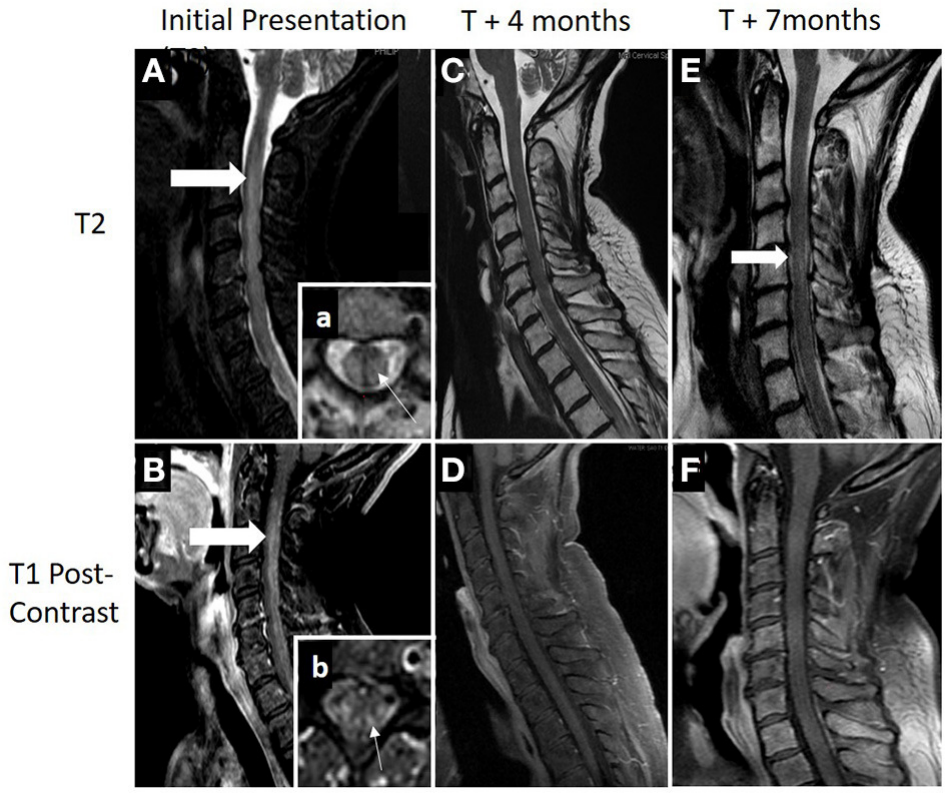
(A,B) MRI demonstrating an longitudinally extensive T2 lesion (top row; thick white arrow) with associated contrast enhancement (bottom row; thick white arrow). Box (a), axial view showing a typical presence of transverse myelitis involving a central lesion, involving more than 2/3 of the cross-sectional area (thin white arrow). Box (b), areas of patchy contrast enhancement concentrated within areas of the posterior cord (thin white arrow). (C,D) Patient had clinical and radiographical improvement at 4 months after diagnosis following acute treatment with plasma exchange, high-dose steroids, and adjuvant chemotherapy. (E,F) Seven months after diagnosis and 1 month following completion of her adjuvant chemotherapy, she was hospitalized again for worsening of lower extremity weakness and pain. Repeat cervical spine MRI showed some worsening T2 signal change within the cord (thick white arrow) with no evidence of contrast enhancement. Acute therapy with plasma exchange and intravenous steroids were given over 5 days followed by rituximab induction (1,000 mg on days 0 and 14). Additional cancer screening with full body PET scan remained negative with clinical relapse.
